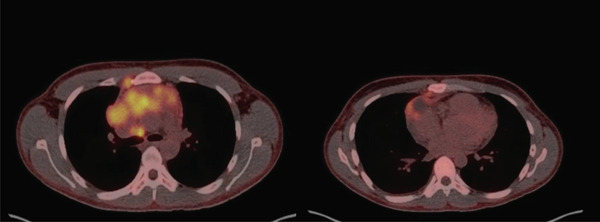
Initial PET‐CT at diagnosis showing a large lobulated 10.2 × 4 cm lobulated FDG‐avid anterior mediastinal mass, loss of fat planes between the mass and major vessels, SUV max 8.5; mass extending into the mediastinum and thoracic inlet. Also with mildly PET‐avid bilateral pleural effusion and pericardial effusion; bilateral paratracheal, anterior jugular, lower jugular lymphadenopathy, anterior pericardiac/diaphragmatic, and right internal mammary lymphadenopathy. T5, L1, and left posterior eighth rib osseous metastasis also evident [5].
